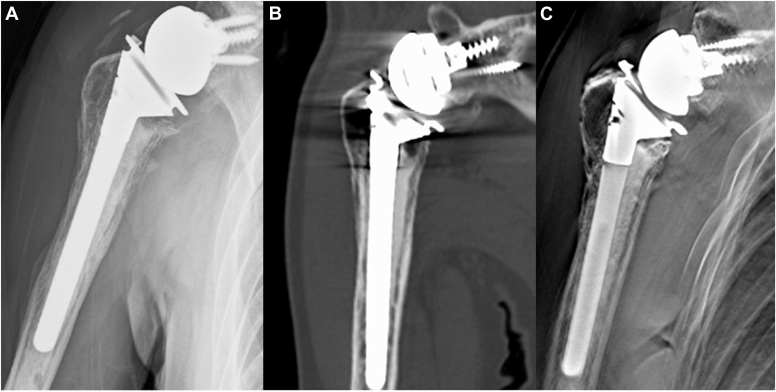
(A) A plain radiograph. No reduction loss was observed. (B) A computed tomography (CT). Metallic artifacts were observed to make evaluation difficult. (C) Tomosynthesis can obtain high-resolution tomographic images by reducing metallic artifacts.
